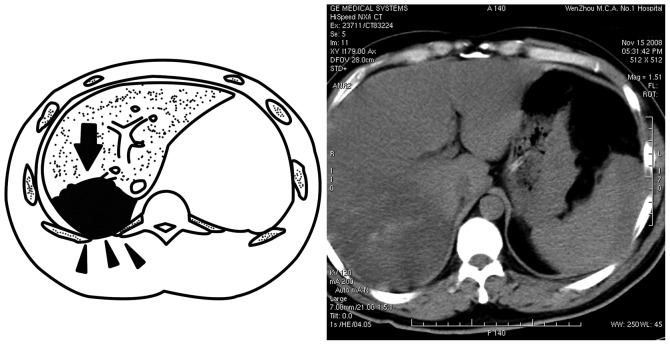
Deceleration injury of the right lobe of the liver. Propelled by huge forces and high speeds (black arrow), the liver moves towards and collides against the posterior abdominal wall (black arrowheads), lacerating this section of the liver. CT film shows a hepatic injury caused in this way by a traffic accident.
